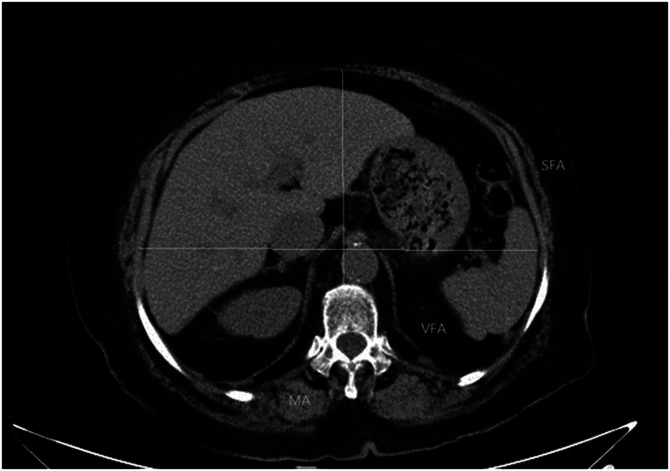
CT at the level of the twelfth thoracic vertebra. MA: Muscle area; SFA: Subcutaneous fat area; VFA: Visceral fat area.
